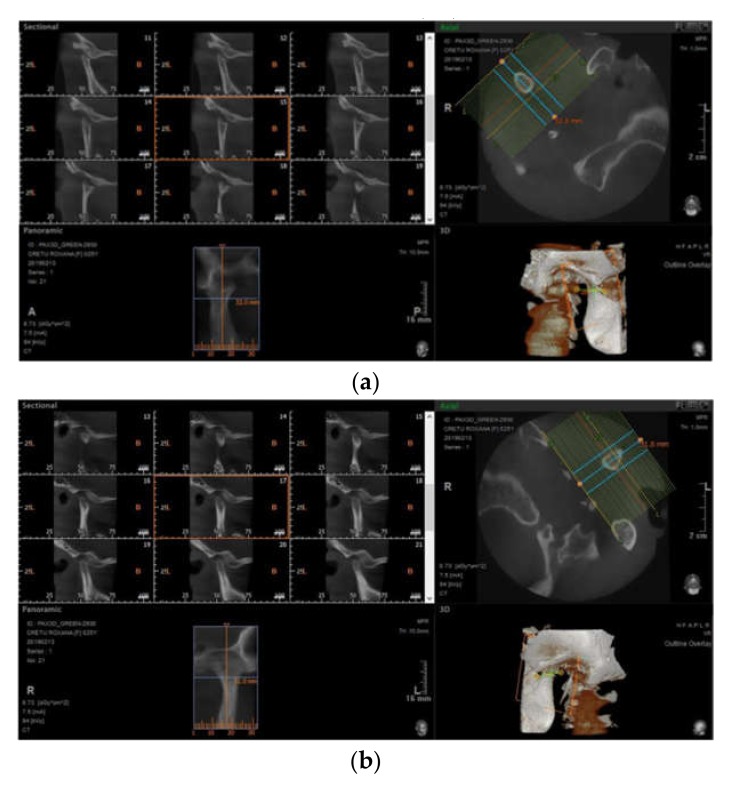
CBCT aspects of the (a) right and (b) left temporomandibular joint (TMJ).
